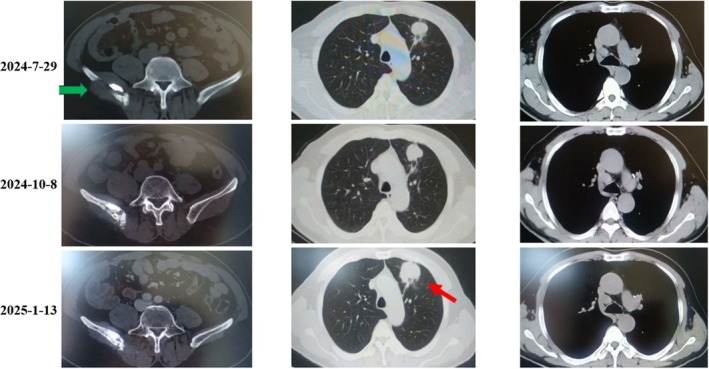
Axial CT images during sixth‐line dual targeted therapy with selpercatinib plus almonertinib combined with local radiotherapy. Left column: Axial pelvic CT showing the right iliac metastatic lesion (green arrow) at baseline (2024‐7‐29), interval progression before radiotherapy (2024‐10‐8), and postradiotherapy follow‐up (2025‐1‐13). Middle column: Axial chest CT (lung window) showing interval enlargement of the primary lung lesion (red arrow) before thoracic radiotherapy and postradiotherapy shrinkage on follow‐up (2025‐1‐13). Right column: Corresponding axial chest CT images in mediastinal window at the same time points.
